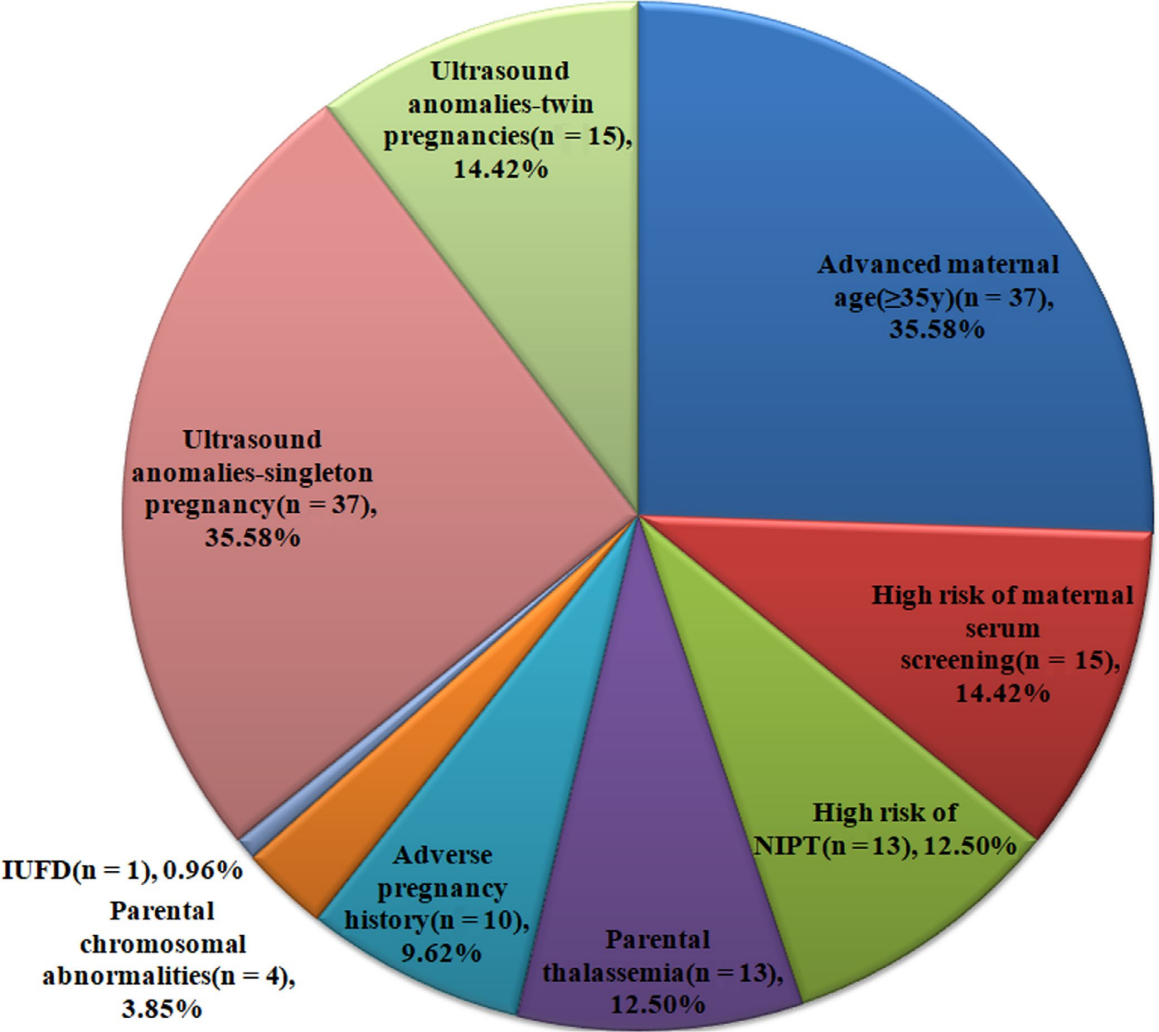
Indications of invasive prenatal diagnosis in foetuses with mosaicism. The sum of the number of cases for different indications exceeds the total number of whole cases (104 cases) because more than one indication may be identified in each case. NIPT, non‐invasive prenatal testing; IUFD, intrauterine foetal death; FGR, foetal growth restriction, n, the number of cases. Parental chromosomal abnormalities include maternal and/or paternal chromosomal abnormalities such as balanced translocation. Ultrasound anomalies include foetal structural defects, soft markers and FGR
